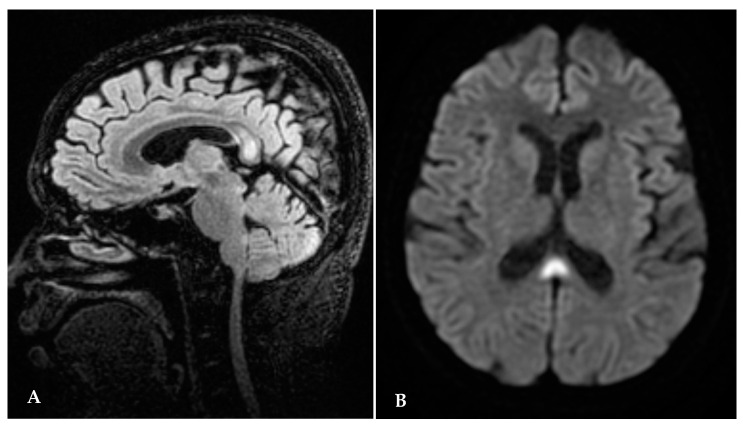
(A,B) Brain MRI after admission: abnormal signal in the splenium of corpus callosum.
